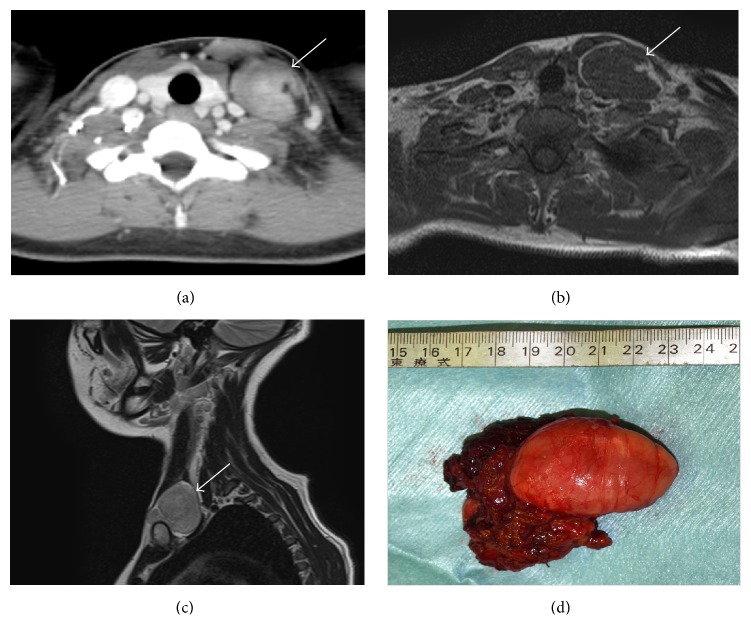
Preoperative imaging findings for Case 2. (a) Contrast-enhanced computed tomography image. T1-weighted axial (b) and T2-weighted sagittal (c) magnetic resonance images. Computed tomography and magnetic resonance images show a tumor with a clear margin and intralesional adipose tissue in the left lower neck. (d) Gross findings of the tumor. The tumor was encapsulated and had a smooth external surface.
